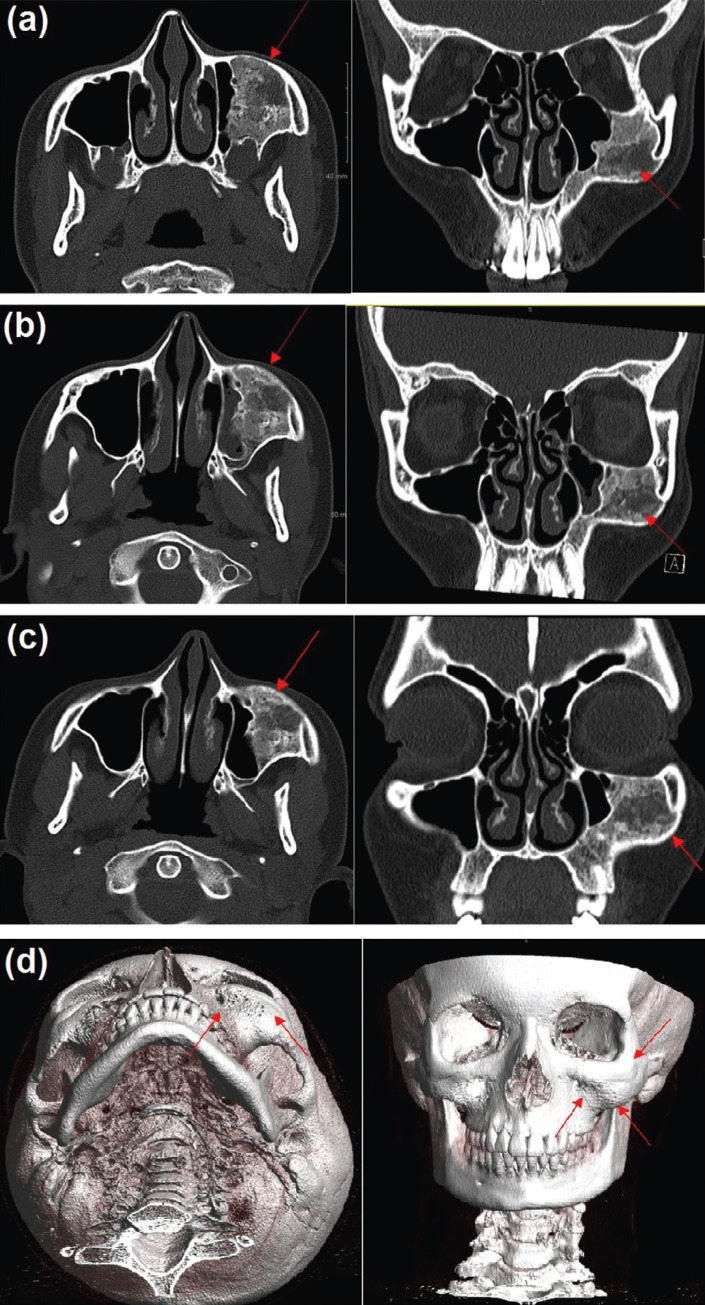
(a) Computed Tomography (CT) Face 2015: Axial and coronal views. Arrows indicating opacification of the left maxillary sinus and bony expansion of left maxilla. Note the “ground glass” appearance of the FD lesion indicated by the arrows, (b) CT Face 2016: Axial and coronal views. Note minimal change from previous scan taken in 2015, (c) CT Face 2017: Axial and coronal views. Minimal to no change in lesion size, (d) 3Dimensional reconstruction of Figure 2 (c). Arrows show size discrepancy between left and right maxilla due to expansion from FD.
